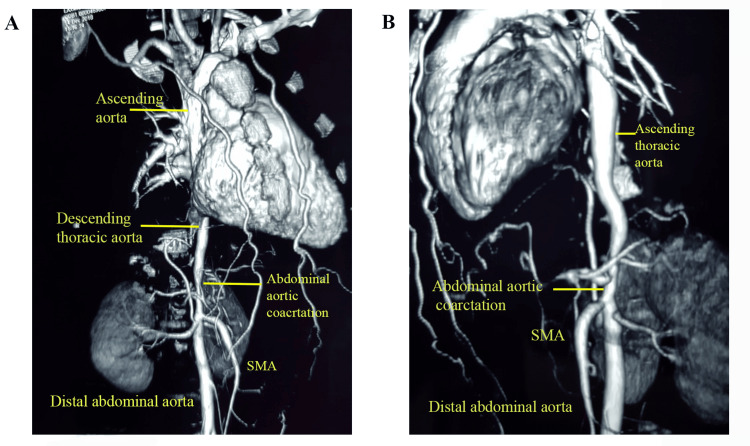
CT aortogram showing coarctation of the abdominal aorta (A) Apical view showing the coarctation segment (8 mm diameter) and distal abdominal aorta (16 mm diameter); (B) Lateral view showing the descending thoracic aorta and the SMA (superior mesenteric artery).
